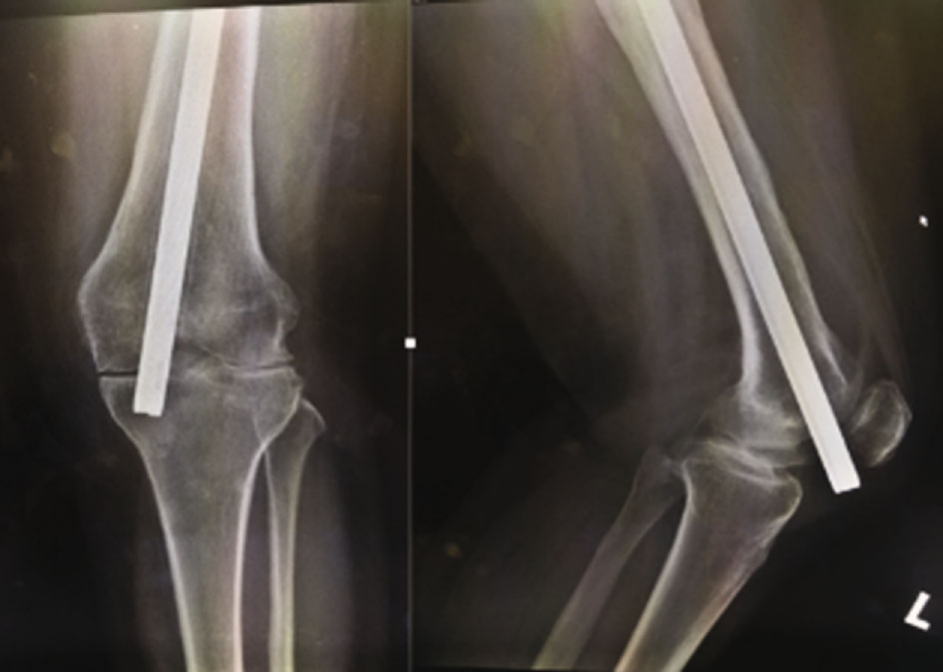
Plain radiograph shows the nail protruded through distal femur.
